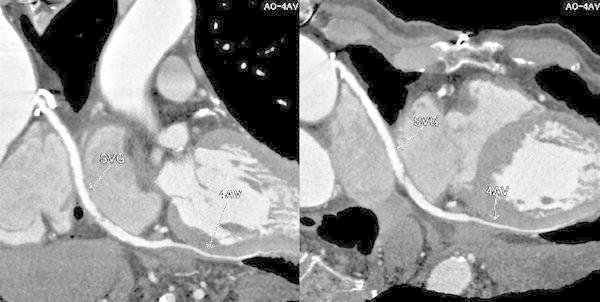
Saphenous vein grafting images of the computed tomographic angiography. A stenosis at the mid portion of the saphenous vein graft
